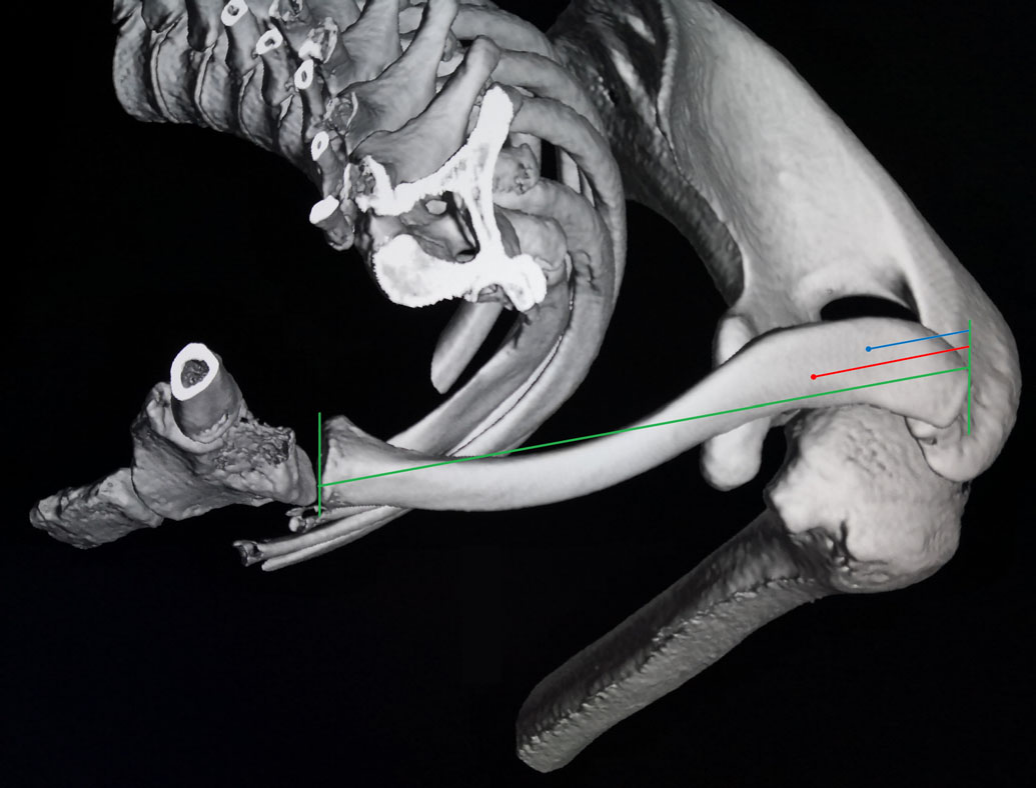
Superior view of 3‐D‐CT reconstruction of a left shoulder showing an example of the planned tunnel location of the conoid (red) and trapezoid (blue) limbs based on anatomic ratios.
